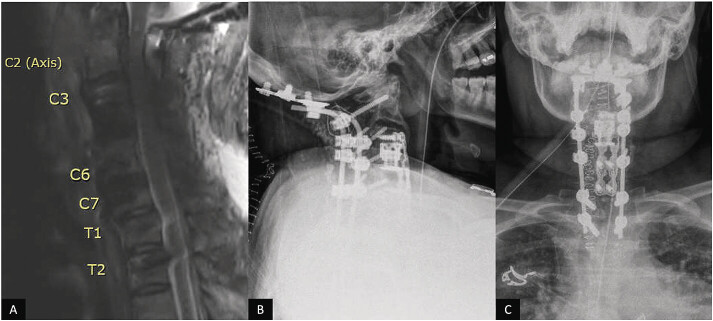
Postoperative T2-weighted MRI (
